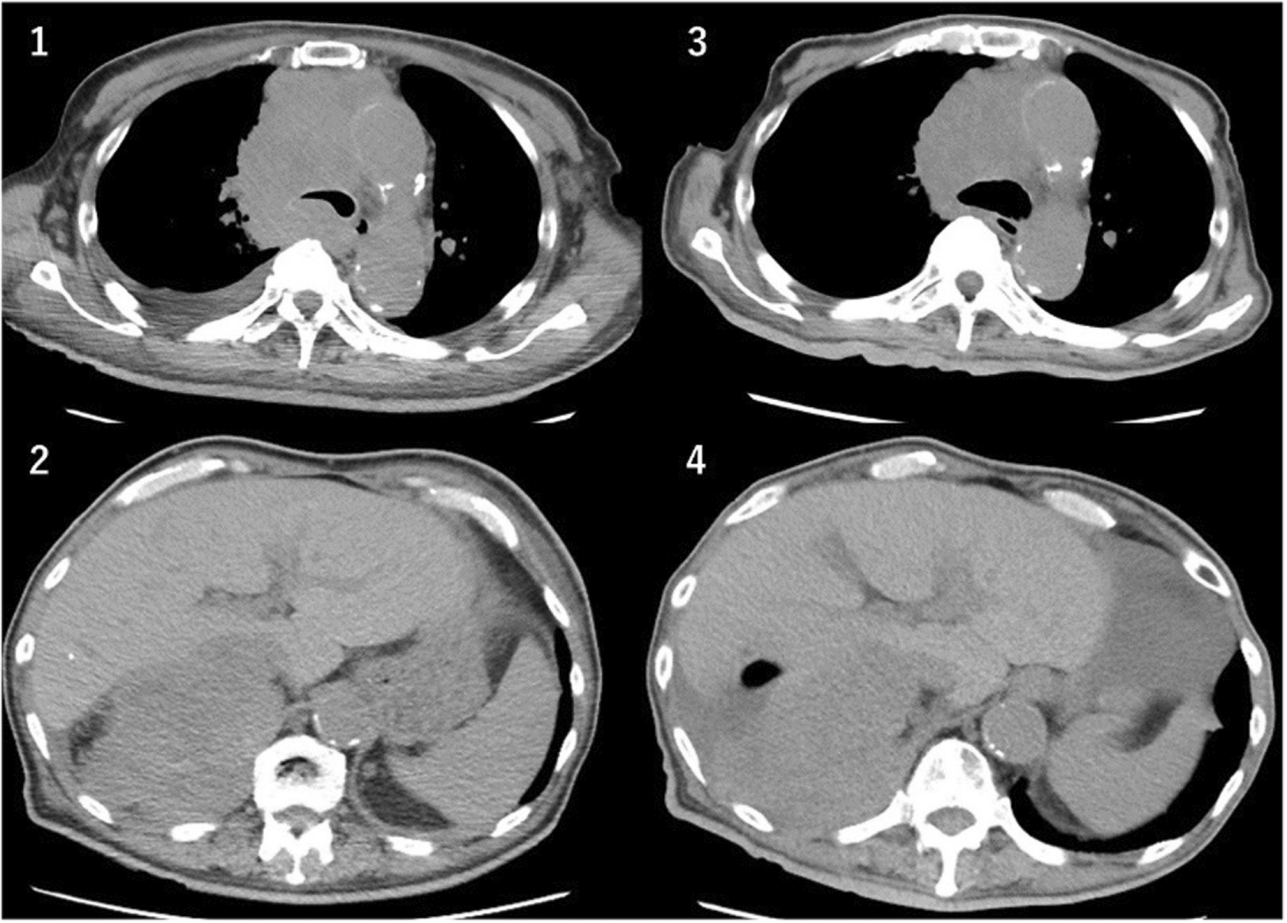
Comparison of pre‐ and post‐therapy CT scans. (1) Mediastinal lymph node metastases at the beginning of treatment; (2) right adrenal gland metastasis at the beginning of treatment; (3) mediastinal lymph node metastases at the end of the treatment second course; and (4) right adrenal gland metastasis at the end of the second treatment course
